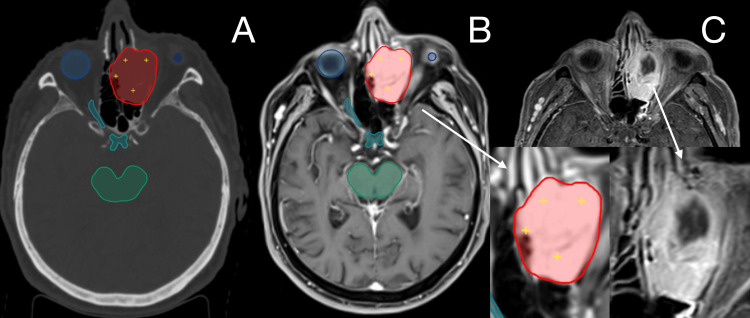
Tumor delineated before radiosurgery in CT (A) and T1 MRI (B), with no progression with central necrosis six months after radiosurgery on T1 MRI (C) for the second patient.
